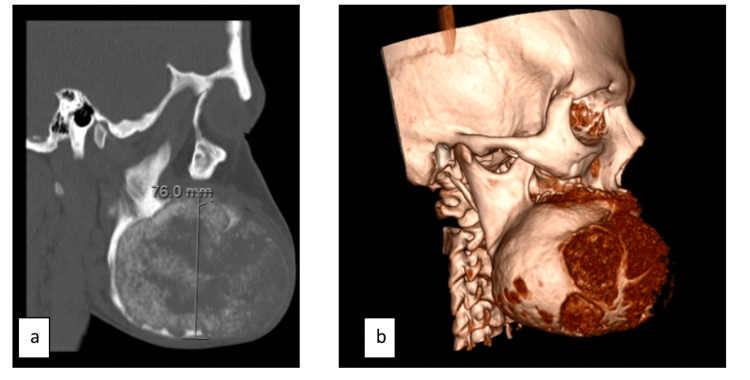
A. Depicts a sagittal CT and B. shows a sagittal reconstruction both demonstrating how the mass contains an internal ground glass calcified matrix. The posterior bony margin of the lesion extends to the anterior margin of the right mandibular ramus.
